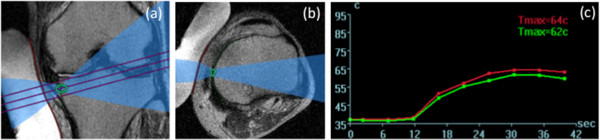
Real-time monitoring. Current sonication spot (green circle) and ultrasound beam pathway are shown in Coronal view (a) and axial view (b). Temperature elevation is monitored by two curves indicated mean (green) and maximum (red) temperature in the spot (c).
